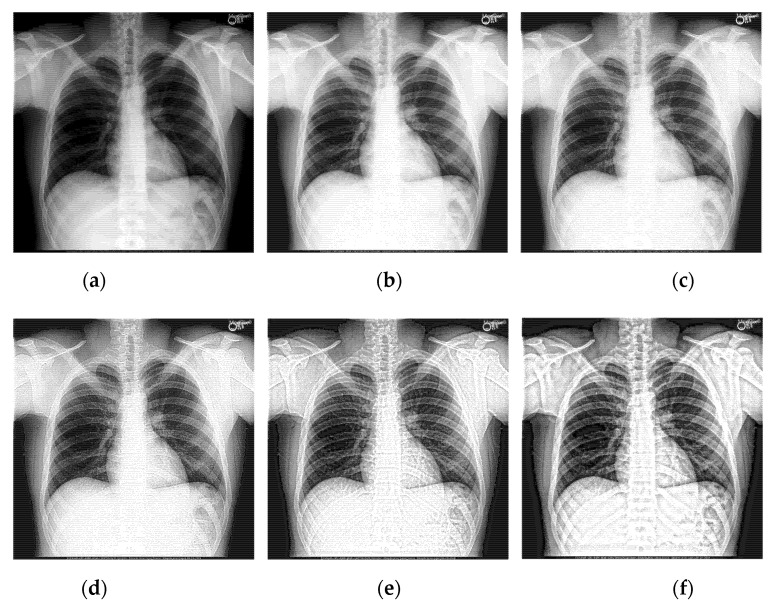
The enhanced effects of different decomposition levels on the X-ray image 2. (a) is the original image, and (b–f) corresponds to the decomposition levels j equal to 1–5.
